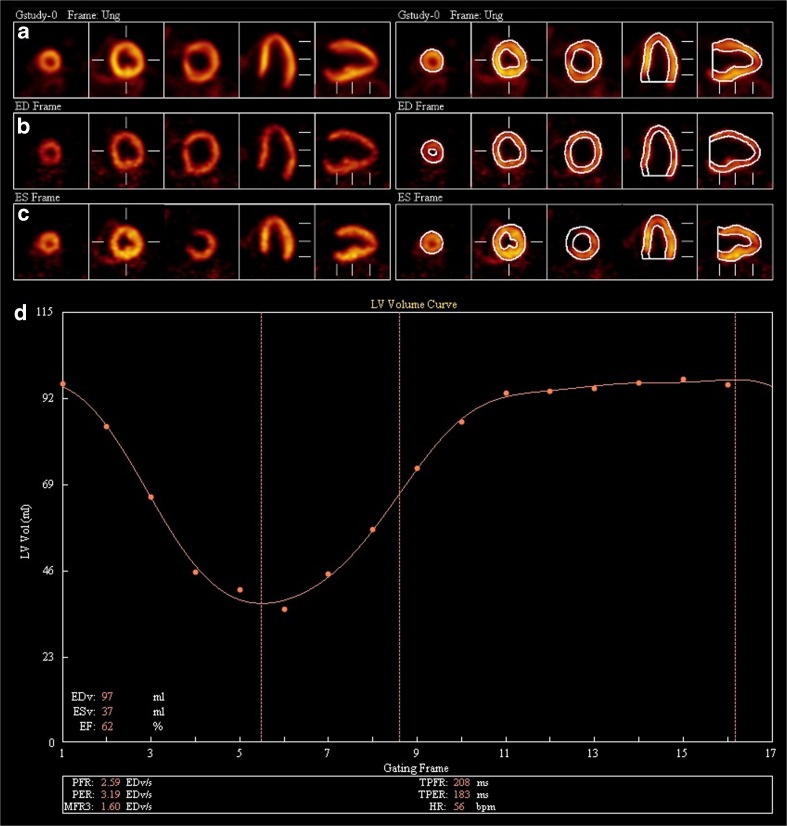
Evaluation of gated PET data using Corridor4DM. *Cardiac PET images of the LV of a patient after a previous PET/CT. Even with fasting using the residual FDG uptake, the image quality was scored 3 (very homogenous myocardial FDG uptake):* Summed frames (A), end-diastolic frames (B), end-systolic frames (C) in three short axes, horizontal long axis and vertical long axis views are shown. The delineation of the left ventricular borders is processed automatically from the PET images (*top right image*) delineation. The time-volume curve of the left ventricle over the different cardiac phases is shown graphically together with the volumetric results in (D). Note that anatomical structures such as the papillary muscles can be depicted better during systole (B) than during diastole (B)
